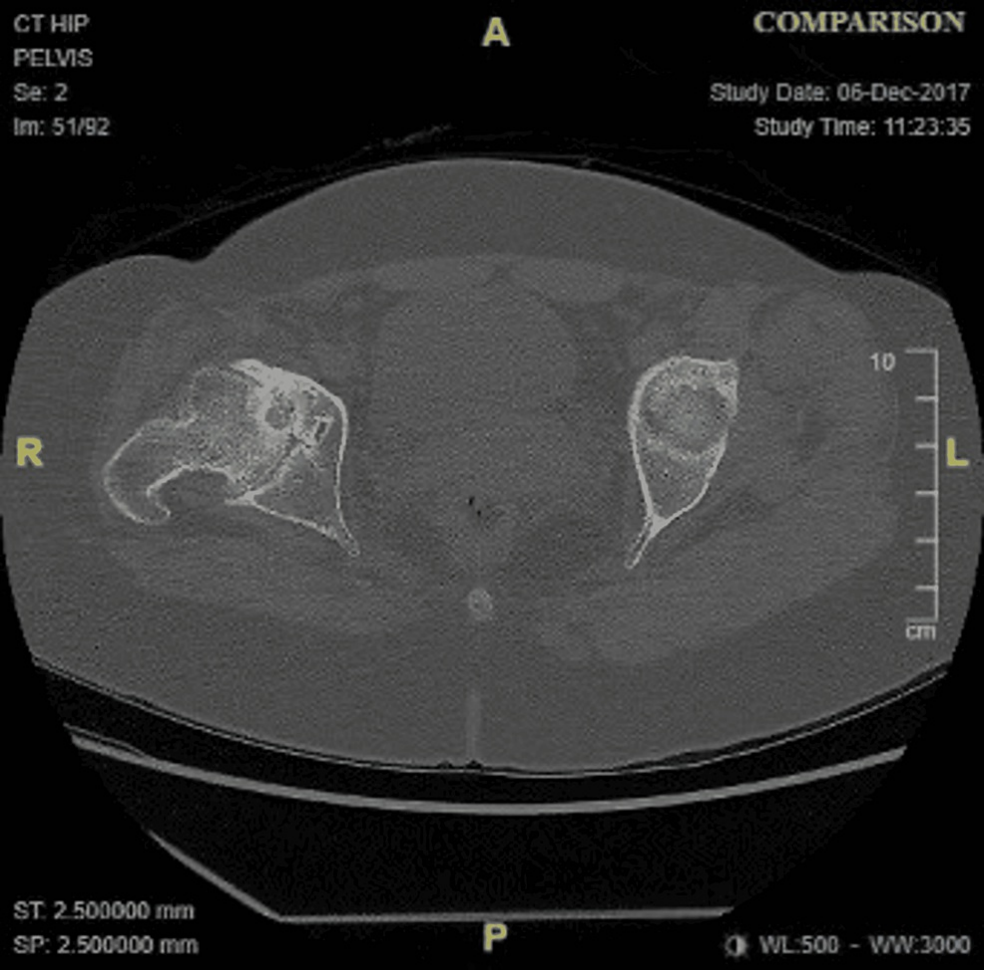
Axial CT showing the hip at the time of presentation.
